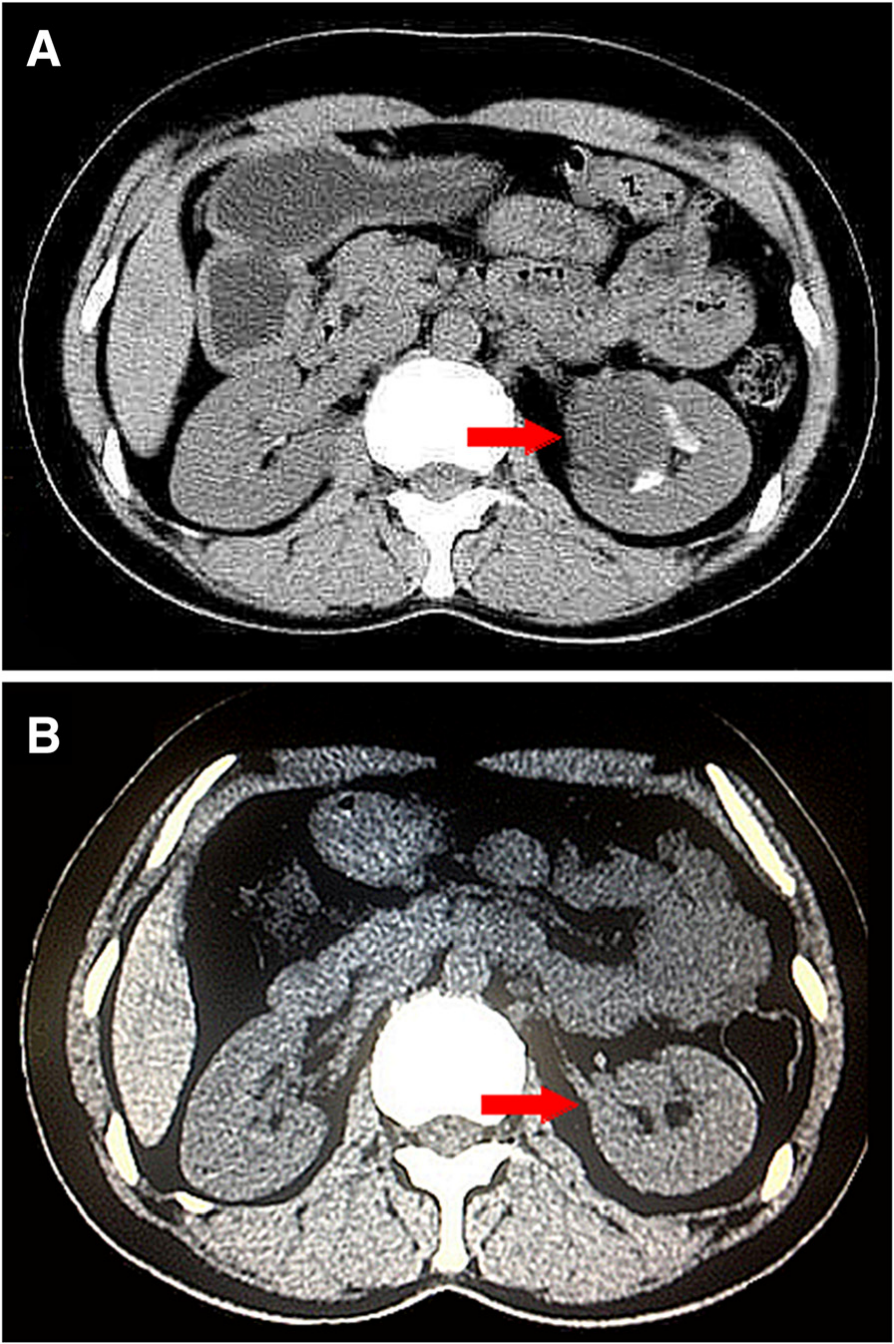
The CT scan showed the parapelvic renal cyst before (Fig. 1a) and 1 month after the operation (Fig. 1b) in a patient (male, 56 years old). The location of the cystis marked by arrows
